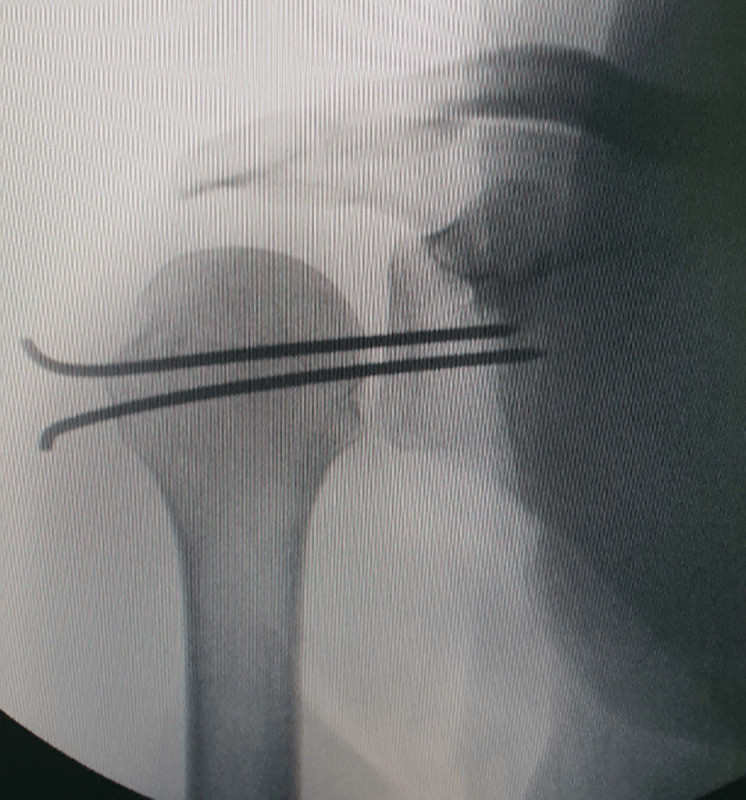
radiographie de face de l´épaule après réduction avec arthrorise gléno-humérale par 2 broches de Kirchner
